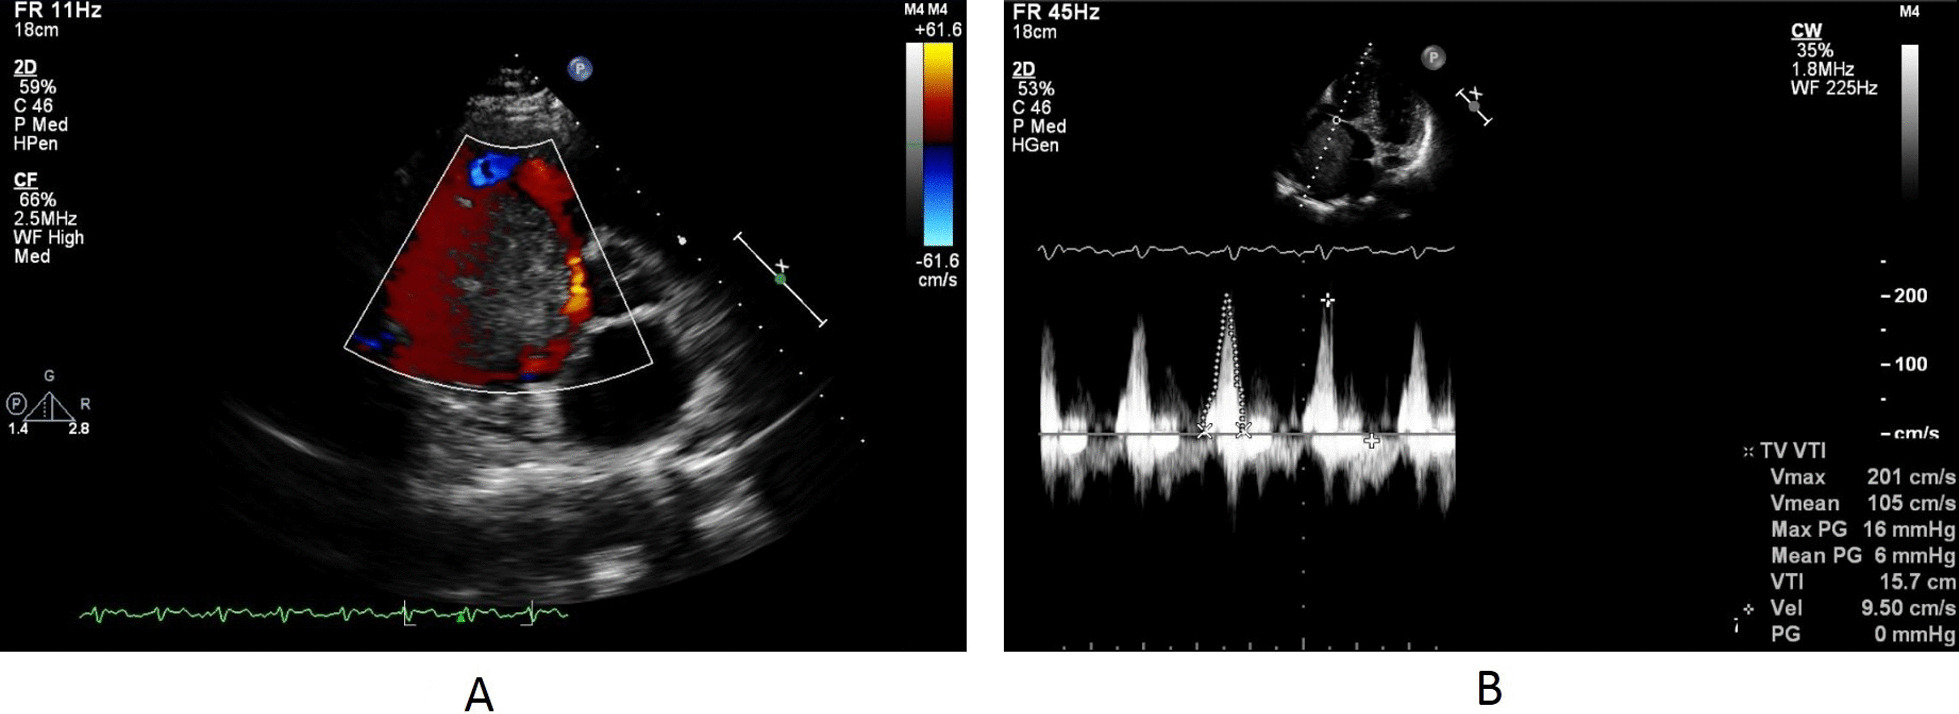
A PSAX view showing the blood flow persisting on the periphery of the mass. B Apical four cavities view with spectral Doppler on tricuspid valve showing a mean gradient of 6 mmHg across the valve and designating a mild to moderate obstruction syndrome
